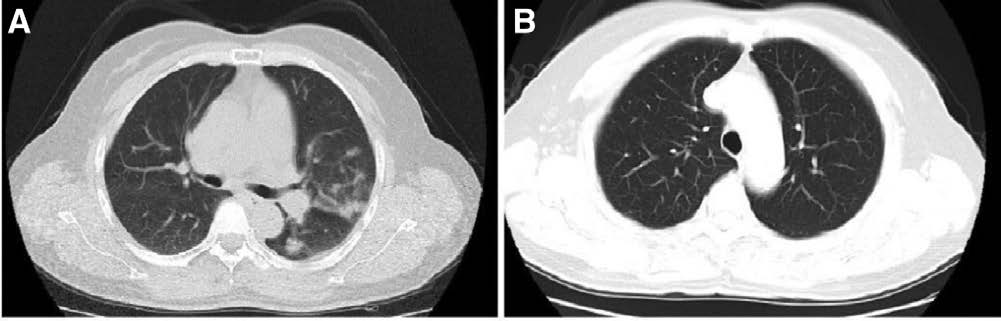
Chest CT of the patients during the treatment. (A) Outpatient checkups; (B) 14 days after azithromycin therapy.
